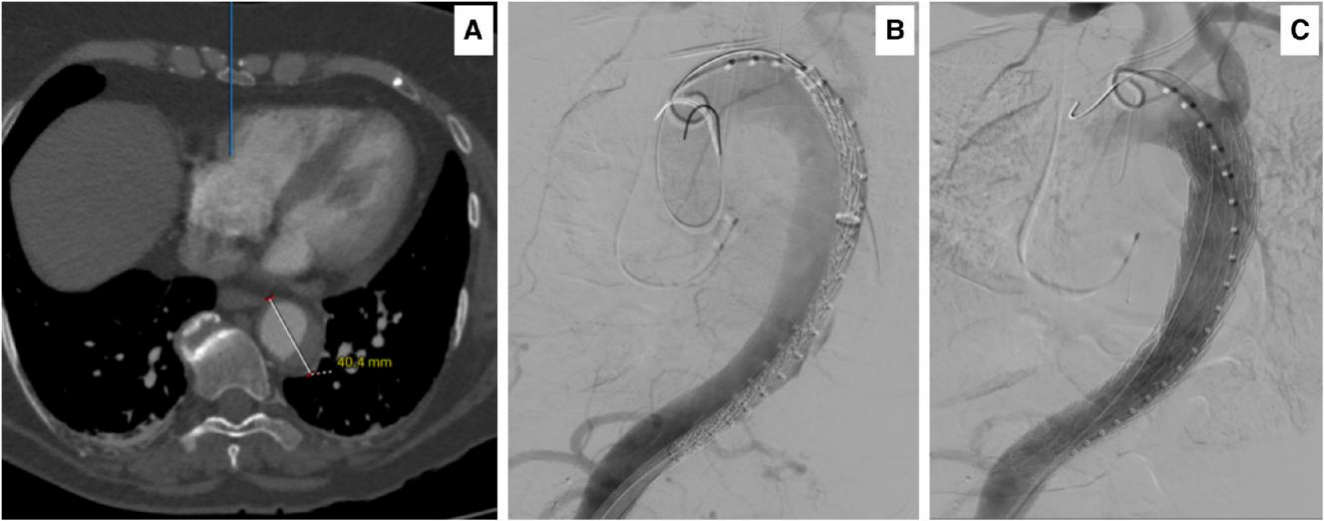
(*A*) Axial computed tomography angiography 18 h post admission demonstrated an increase in the size of acute intra-mural haematoma and aorta to 40.4 mm. (*B*) Intra-operative digital subtraction angiography, documenting the leak at the level of T9–T10 and CTAG in position before deployment. (*C*) Complete intra-operative digital subtraction angiography, documenting good positioning of the thoracic endovascular aortic repair CTAG graft and excluding the acute intra-mural haematoma. Note the patent left subclavian artery and celiac axis.
